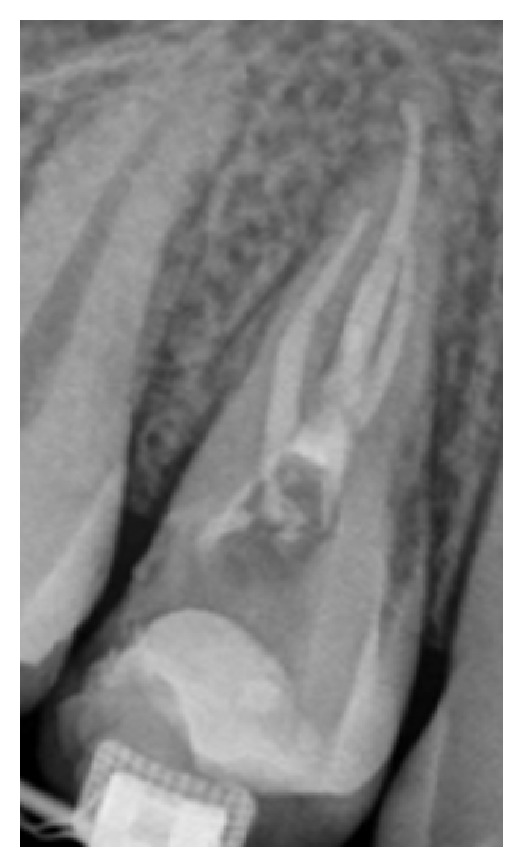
Periapical radiography of tooth 14 after 12 months, revealing no presence of failure in filling of root canals or periapical lesions.
